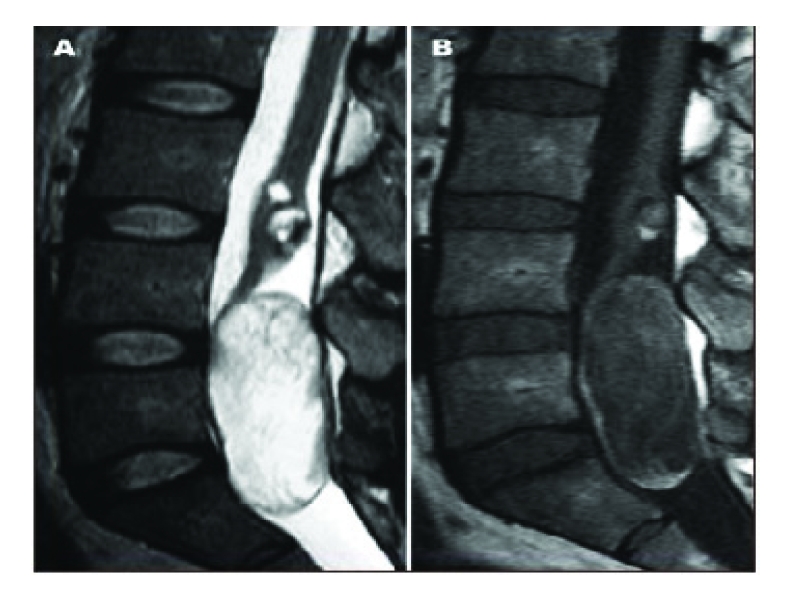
MRI image of cauda equina epidermoid cyst: note anterior displacement of the medullarycone
